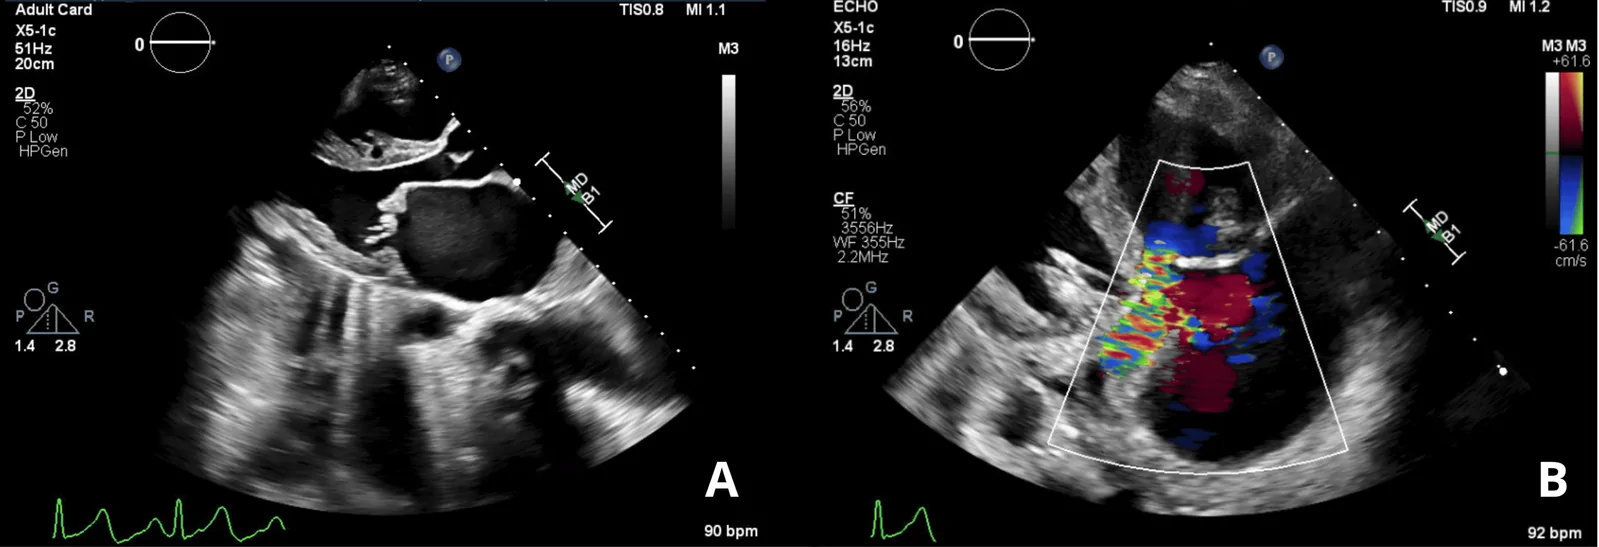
Transthoracic echocardiography in the parasternal long-axis view demonstrating mitral stenosis A: two-dimensional echocardiographic image demonstrating thickening and calcification of the mitral valve leaflets with restricted opening, resulting in the characteristic "hockey stick" deformity; B: color Doppler flow mapping demonstrating accelerated turbulent transmitral flow with aliasing across the mitral valve into the left ventricle, consistent with a markedly elevated transmitral gradient due to significant valvular obstruction.
